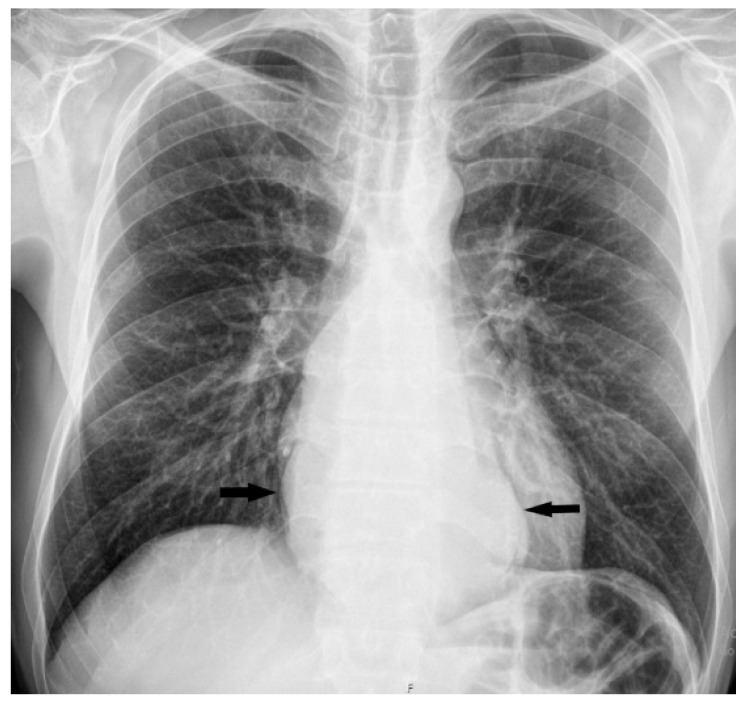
Upright posteroanterior (PA) chest radiograph showed a well-defined, oval soft-tissue opacity located retrocardially (black arrows) in a 45-year-old man who came to our institution with progressive symptoms of dysphagia and anemia, which had been present for about eight months. He presented with Hgb levels of 64 g/L, and his disease history revealed several episodes of hematemesis and melena within the two months prior to admission. Over this period, he unintentionally lost around 20 kg. Esophagogastroduodenoscopy (EGDS) was performed and showed a large, vegetative mass starting at 32 cm from the upper incisors, with almost complete obstruction of the esophageal lumen, though the endoscope could still pass. There was a significant amount of hemorrhagic content inside the lumen of the esophagus and stomach. The mass extended all the way to the cardia, where biopsies were taken. In retroversion, a bulky, oval-shaped polypoid lesion was seen protruding into the gastric lumen from the cardia, roughly 10 cm in size. After EGDS, a multislice CT scan (MDCT) confirmed a large, irregular, partly necrotic mass narrowing the esophageal lumen, spreading caudally across the gastroesophageal junction and infiltrating the cardia and proximal stomach. The tumor was in contact with the pericardium with a lateral impression on the mediastinal pleura, but without radiological signs of infiltration (Figure 2).
